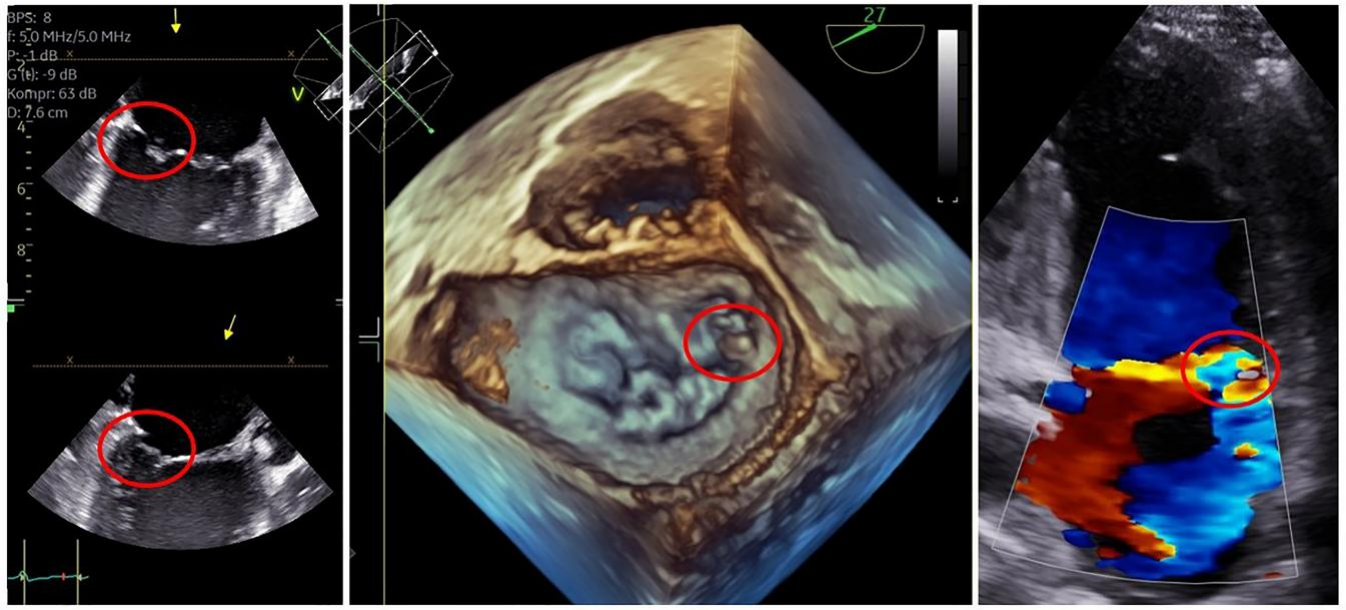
Multimodal transoesophageal echocardiographic visualization of flail posterior mitral leaflet with chordal rupture following microaxial flow pump support. Two-dimensional (left and right panels) and three-dimensional (middle panel) transoesophageal echocardiographic images obtained after explantation of the microaxial flow pump, demonstrating flail posterior mitral leaflet (PML) with chordal rupture. The rupture site is highlighted by circles across all modalities. The middle panel presents an *en face* 3D view of the mitral valve from the left atrial perspective, clearly delineating the disrupted subvalvular structure. The right panel, using colour Doppler imaging, visualizes the associated severe mitral regurgitation.
